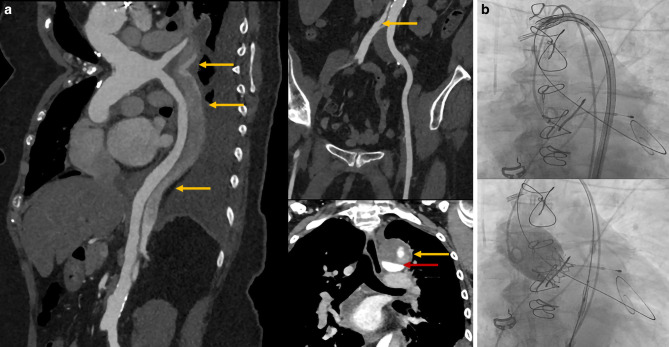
a Preprocedural computed tomography scans showing chronic aortic dissection extending into left proximal carotid artery and left subclavian artery, left renal artery and both common iliac arteries (all indicated by *yellow arrows*). Red arrow indicates true lumen of aortic arch. b New valve was passed through dissected aortic arch as operators gave traction on guide wire to make sure inner curve was followed. New valve was implanted without any complications
